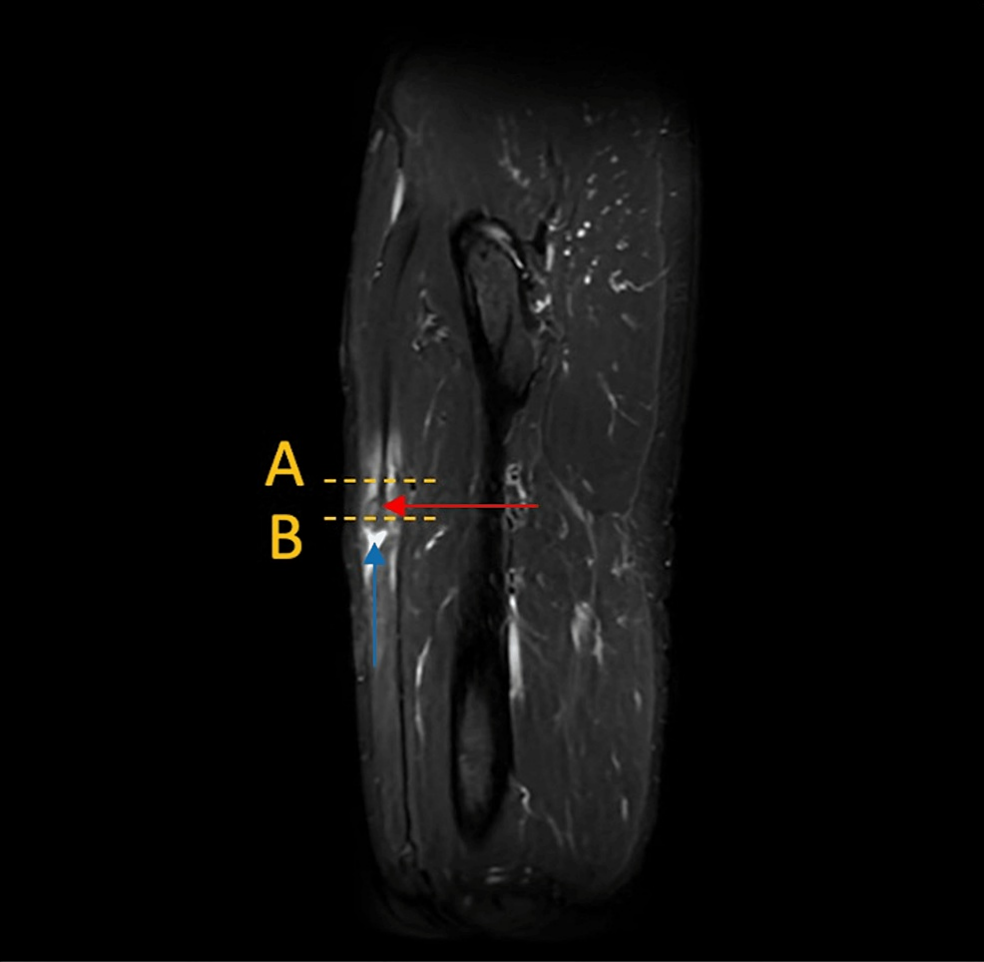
Sagittal STIR MRI demonstrating the combination of tearing (blue arrow) and up to 23 mm retraction of the inner muscle (red arrow). This is analogous to a finger in a glove, also known as an intramuscular degloving of the rectus femoris. STIR, Short Tau Inversion Recovery; MRI, magnetic resonance imaging
